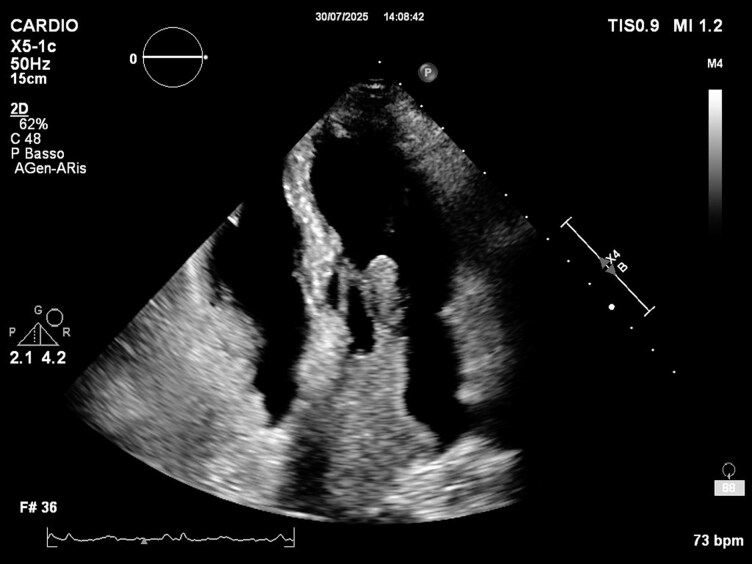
Transthoracic echocardiographic four-chamber view showing the intra-atrial mass prolapsing into the left ventricle.
